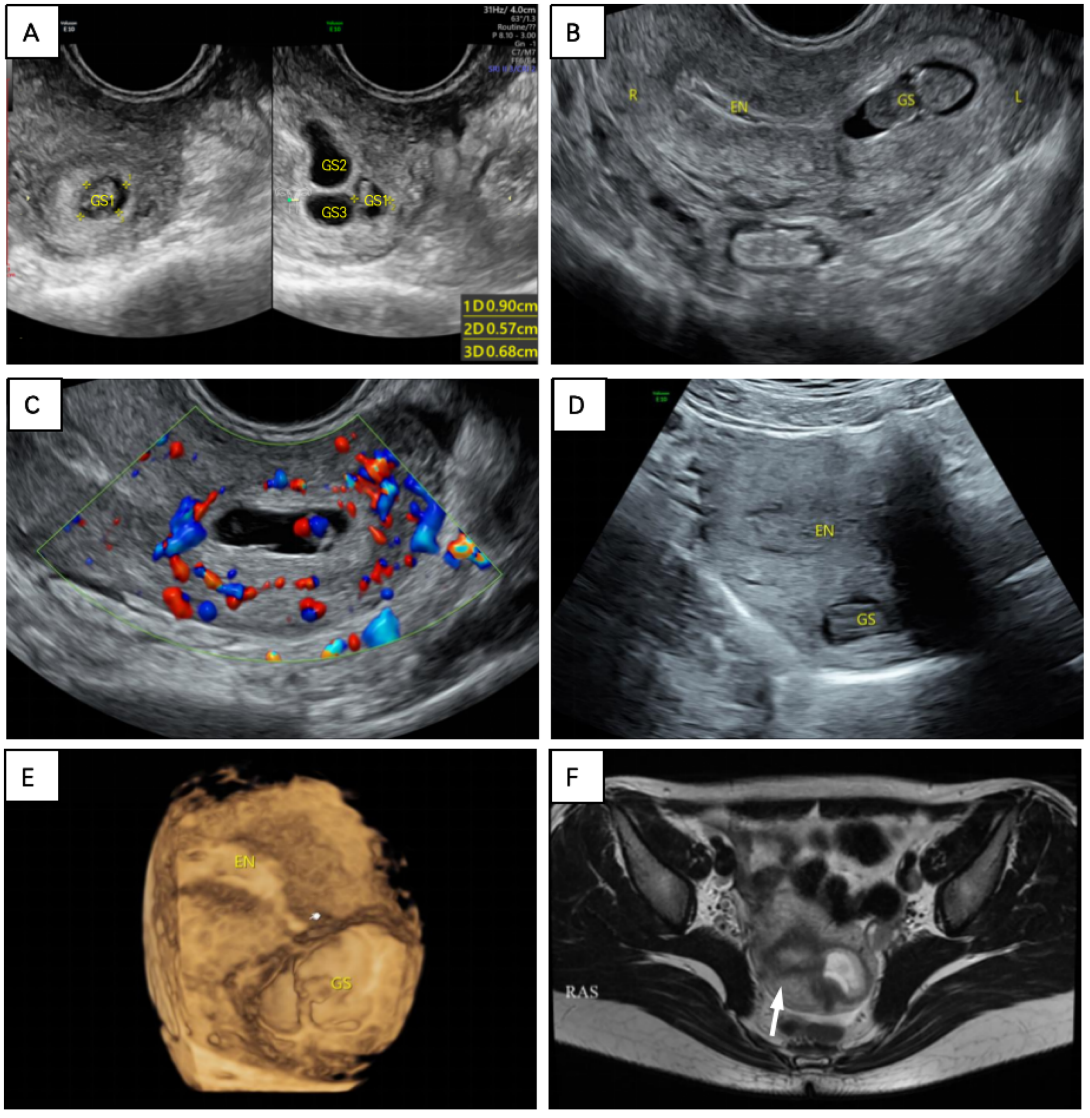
(A) Initial transvaginal ultrasound revealed three hypoechoic areas, one with internally visible yolk sacs and embryonic buds. (B) The gestational sac at the base of the uterus was observed again on transvaginal ultrasound 1 week after curettage. (C) CDFI appeared to detect myometrial blood flow between the sac and the endometrium. (D) Transabdominal ultrasound and (E) three-dimensional ultrasound imaging revealed that the sac was outside the endometrium (The white finger indicates the endometrial end of the uterus). (F) On the T2-weighted MRI sequence, where the white arrow points to, the high signal area is the endometrium, the surrounding low signal area is the union zone, and the left high-signal gestational sac is located outside the endometrium.
